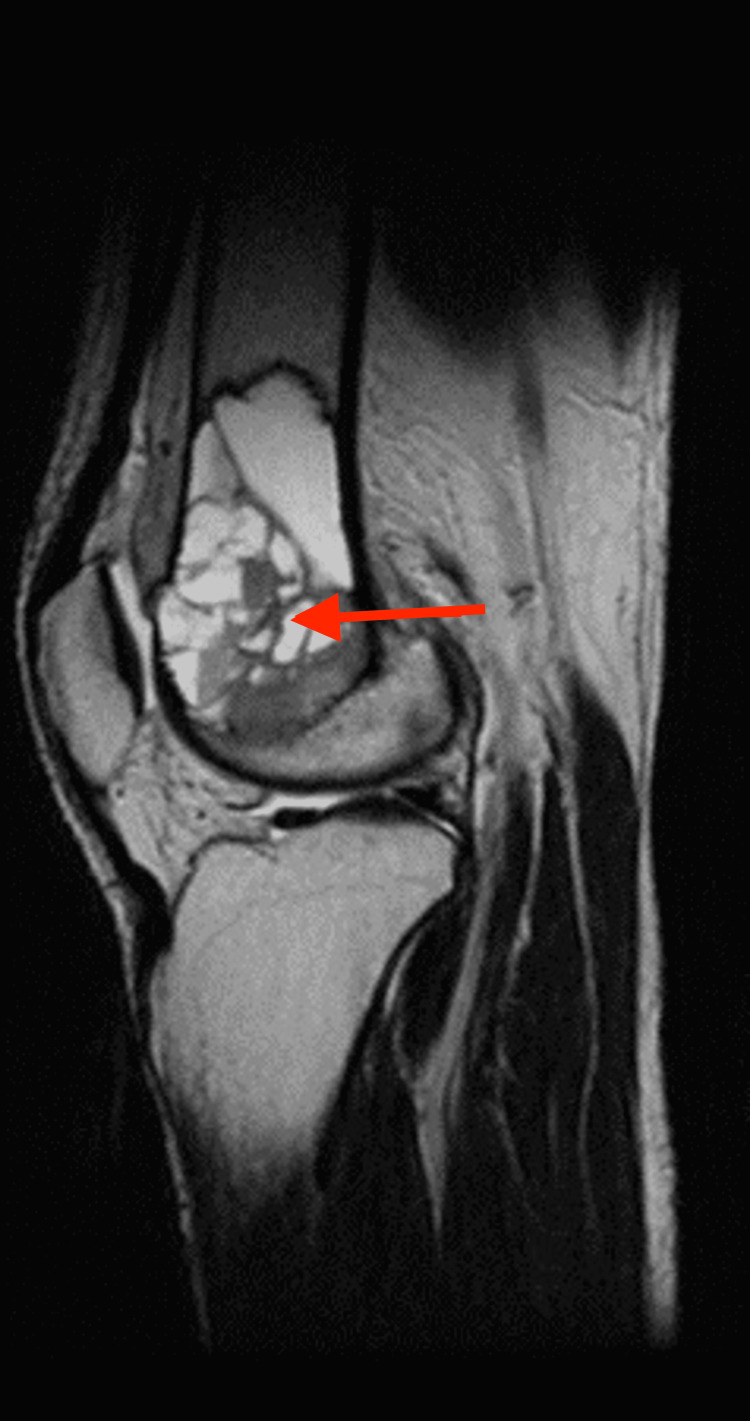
MRI of the left knee showing well-defined multiloculated expansile altered signal intensity mass lesion at the distal end of femur appearing hyperintense on T2WI, variable intensity on T1WI, showing fluid-fluid levels within the locules with areas of blooming on T2 and hyperintensities in T1WI suggestive of hemorrhagic components
